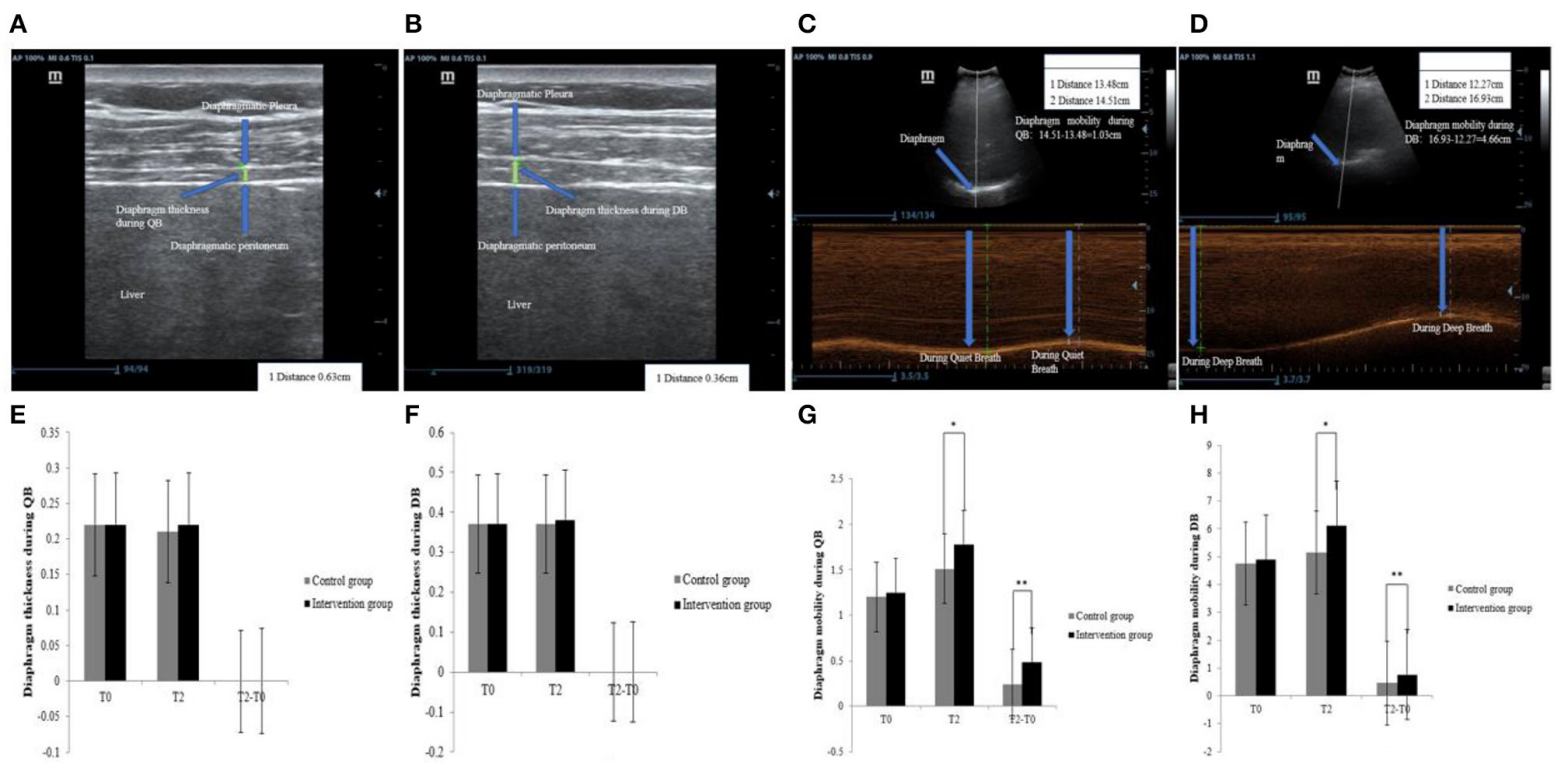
Ultrasound and comparison of diaphragm thickness and mobility. (A) diaphragm thickness during QB, (B) diaphragm thickness during DB, (C) diaphragm mobility during QB, (D) diaphragm mobility during DB, (E) comparison of diaphragm thickness during DB, (F) comparison of diaphragm mobility during QB, (G) comparison of diaphragm mobility during QB, (H) comparison of diaphragm mobility during DB. QB, Quiet Breath; DB, Deep Breath; *Significant differences between groups (*P* < 0.05); The difference between groups was highly significant (*P* < 0.01).
